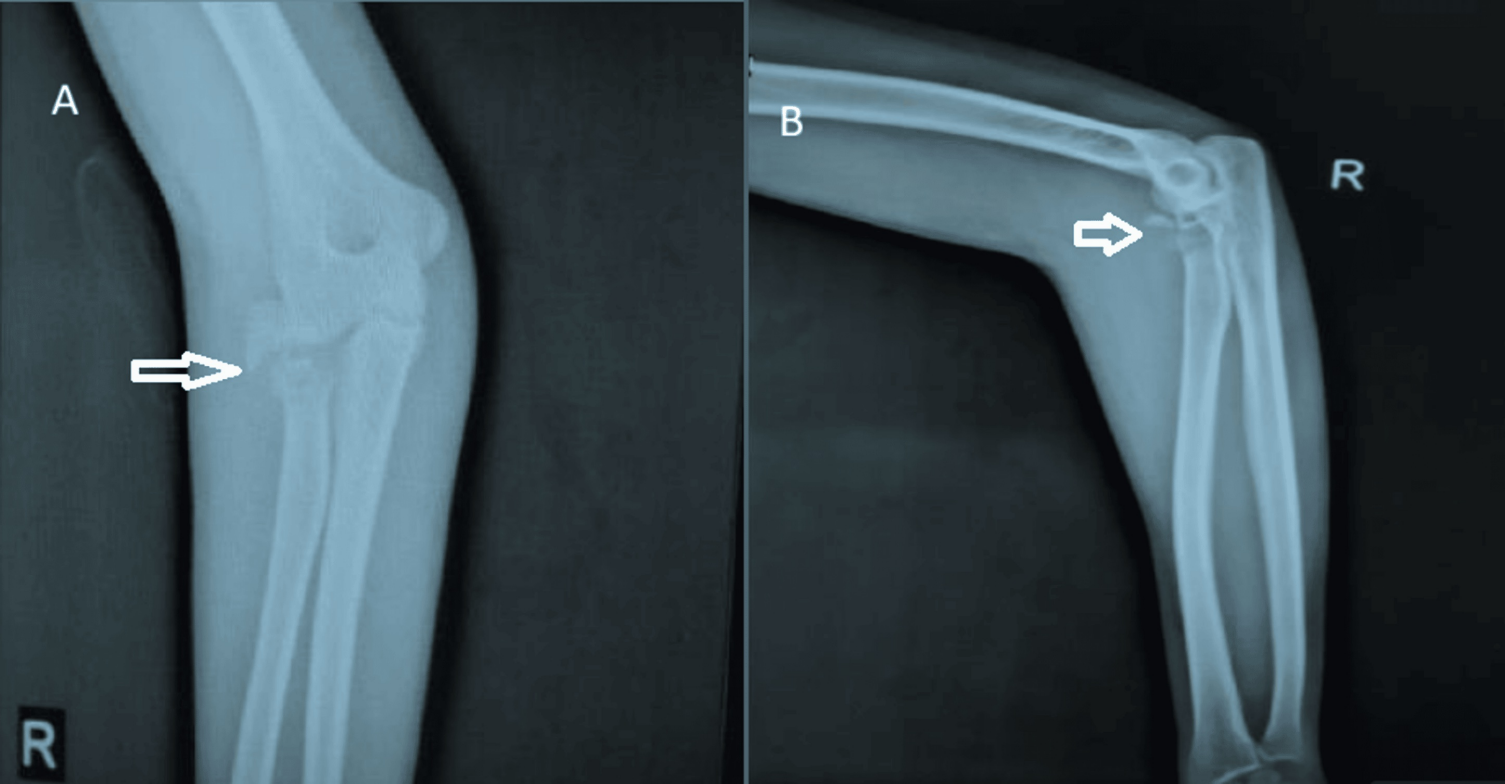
A) Preoperative radiograph showing the anteroposterior (AP) view of the right elbow with Mason type III radial head fracture. B) Preoperative radiograph showing the lateral view of the right elbow with Mason type III radial head fracture.
